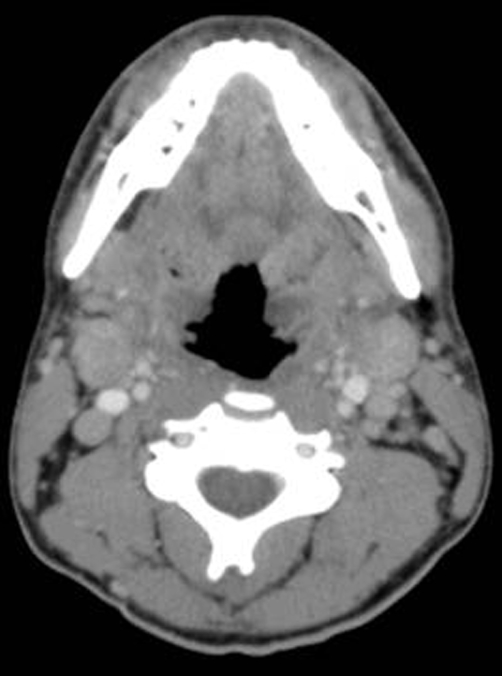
CT scan of head and neck. Note cervical lymphadenopathy.
